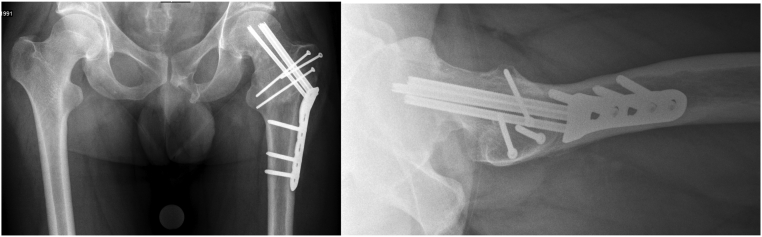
AP pelvis and cross-table lateral hip radiographs after open treatment including bone grafting, cam reduction and revision osteosynthesis with a Synthes© Pediatric hip plate.
